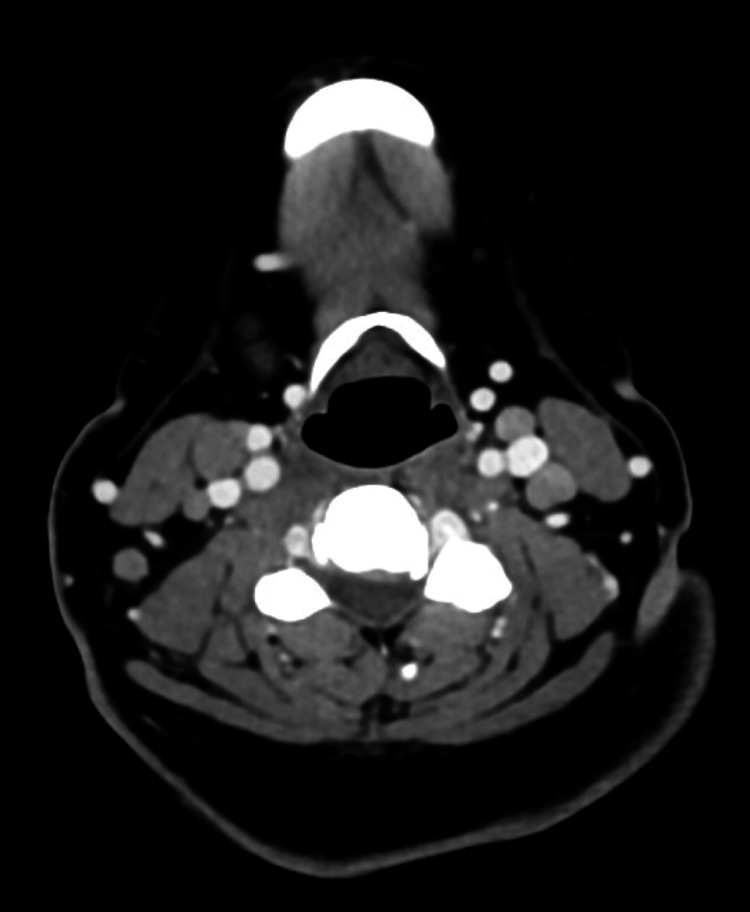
CT neck/soft tissue with contrast showing bilateral anterior and posterior cervical lymphadenopathy CT: computed tomography
